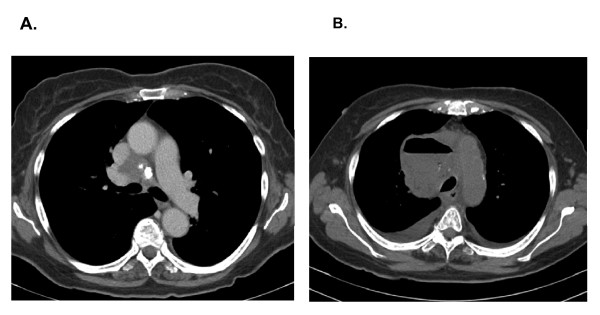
Computed Tomography Scan of Mediastinal Pathology. A. The patient had a preoperative CT scan that revealed mediastinal adenopathy. B. Two weeks after EBUS and transbronchial needle aspiration, the patient presented with a complex mediastinal abscess.
